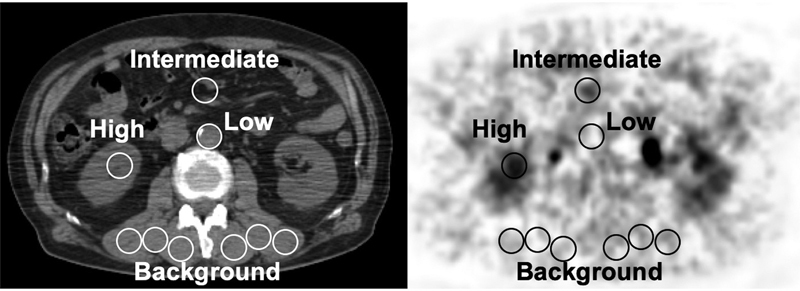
Regions of interest placed on a clinical positron emission tomography/computed tomography image.
